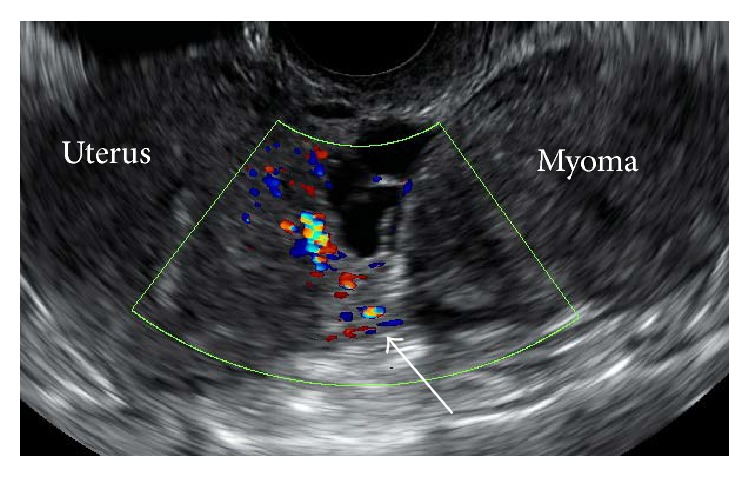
Transvaginal color Doppler ultrasonography showing a pedunculated myoma connected to the uterine body. The blood flow was interrupted at the base of the myoma pedicle (arrow), suggesting the occurrence of torsion.
